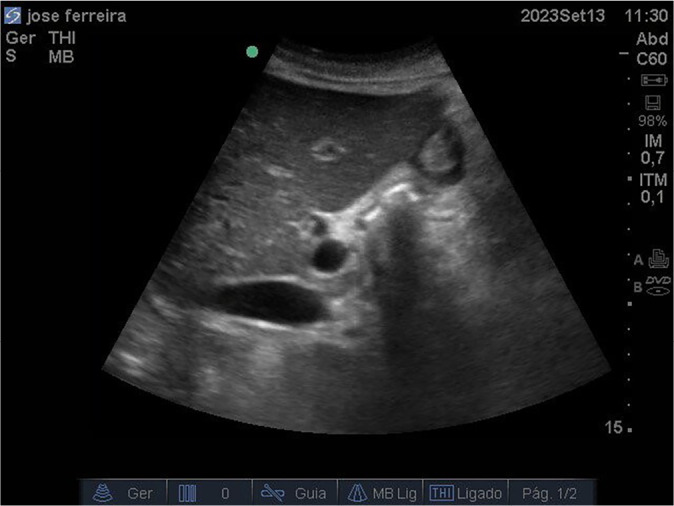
Gastric antrum ultrasound image on intensive care unit day 30
